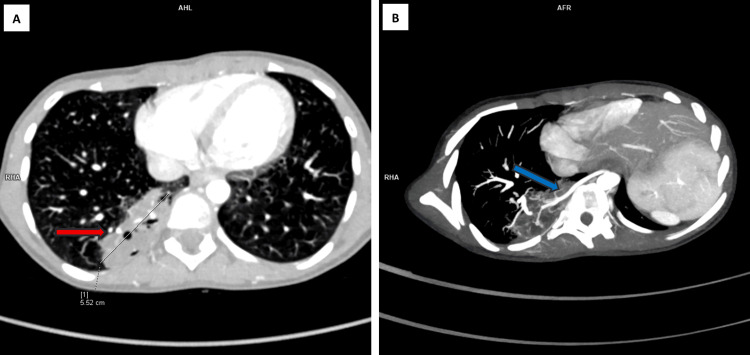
CECT angiographic images of the chest and upper abdomen. (A) CECT (axial section) demonstrating a cavitary lesion in the posterior basal segment of the right lower lobe (red arrow) measuring approximately 5.5 cm lacking any obvious bronchial communication. (B) CT angiographic reconstruction showing the anomalous systemic arterial feeder (blue arrow) arising from the descending thoracic aorta supplying the lesion CECT: contrast-enhanced computed tomography; CT: computed tomography; AHL: axial high-level view; RHA: right-handed acquisition; AFR: arterial flow reconstruction
